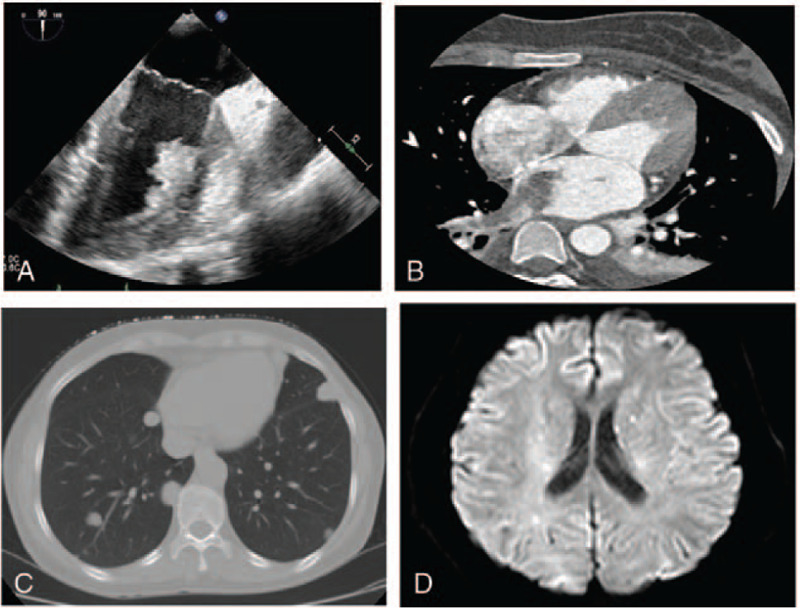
Echocardiogram and pulmonary angiography of Patient. Transesophageal echocardiography (Fig. 2A) and pulmonary angiography revealed a thrombus (Fig. 2B) in the right inferior pulmonary vein contiguous to left atrium and ventriculus. Computed tomography scan of the chest revealed numerous small metastatic lesions (Fig. 3C). Magnetic resonance imaging of the head revealed multiple infarcts and hemorrhages in the brain (Fig. 3D).
